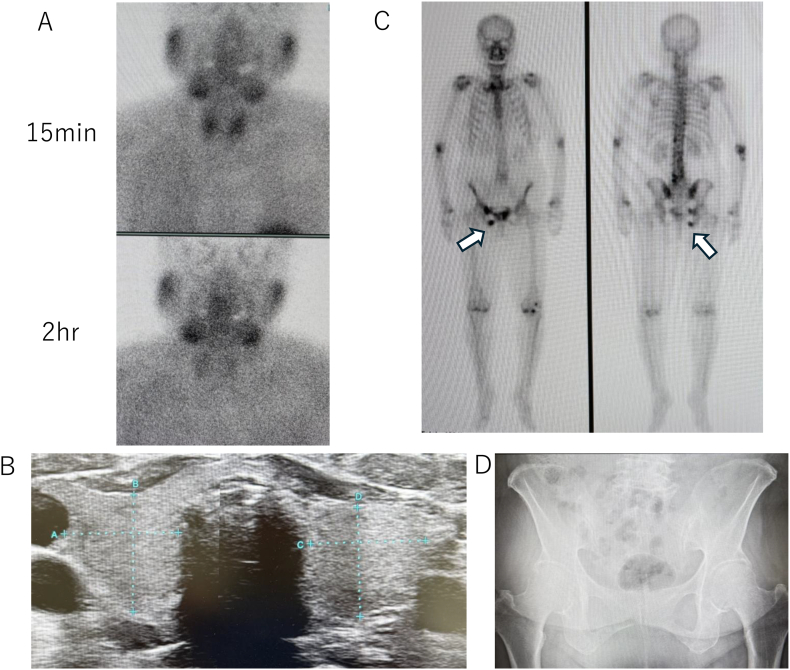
Imaging studies. (D) Pelvic radiography demonstrating sclerotic changes at the pubic fracture site (arrow). Initially, these findings suggested the possibility of Paget's disease; however, they were ultimately determined to be indicative of fracture healing.
